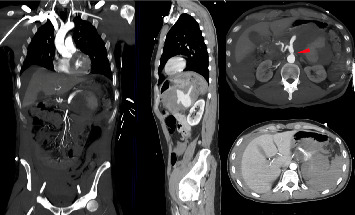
Contrast enhanced computed tomography scans. The red arrow indicates dissection of the celiac tripod at the origin.
